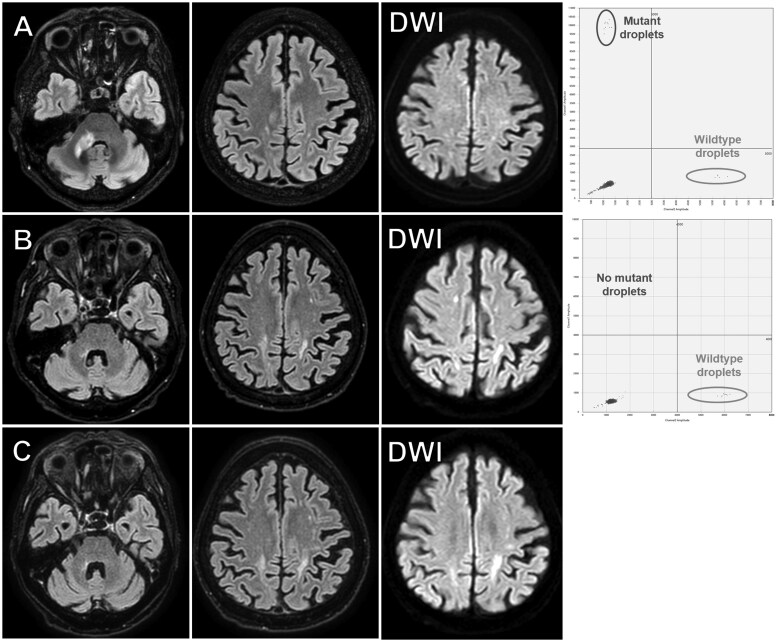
MR images (FLAIR and DWI) and the ddPCR result of patient #8 before treatment (A) and 5 months after treatment (B). New hyperintense lesions on FLAIR and DWI were observed in the bilateral parietal deep white matter but *MYD88* L265P-mutant droplets were not observed (B). MR images (FLAIR and DWI) 6 months after the initial observation of the parietal lesions (C). The lesions have primarily remained unchanged and recurrence of PCNSL was considered unlikely. ddPCR, droplet digital PCR; DWI, diffusion-weighted image; PCNSL, primary central nervous system lymphoma.
